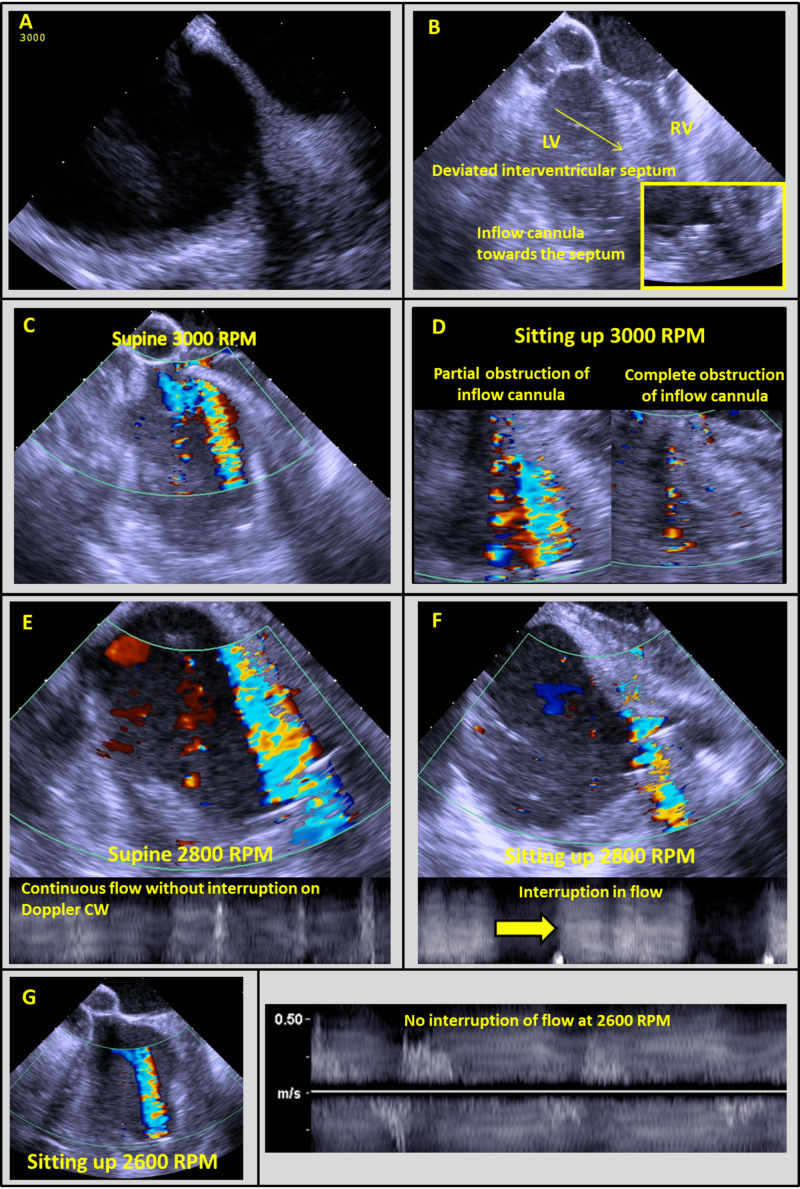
Intracardiac echocardiographic evaluation of HVAD inflow obstruction Initial evaluation demonstrating midline interatrial septum (A) and rightward interventricular septum as well as septal-oriented inflow cannula (B). Supine imaging at 3000 RPM showed normal flow (C), which was obstructed after sitting (D). Similar results were seen at 2800 RPM and inflow cannula CW demonstrated cessation of flow with sitting (E, F). At 2600 RPM in the setting position, no diminishment in the flow was seen by color flow or CW interrogation (G). RPM - revolutions per minute; CW - continuous wave.
